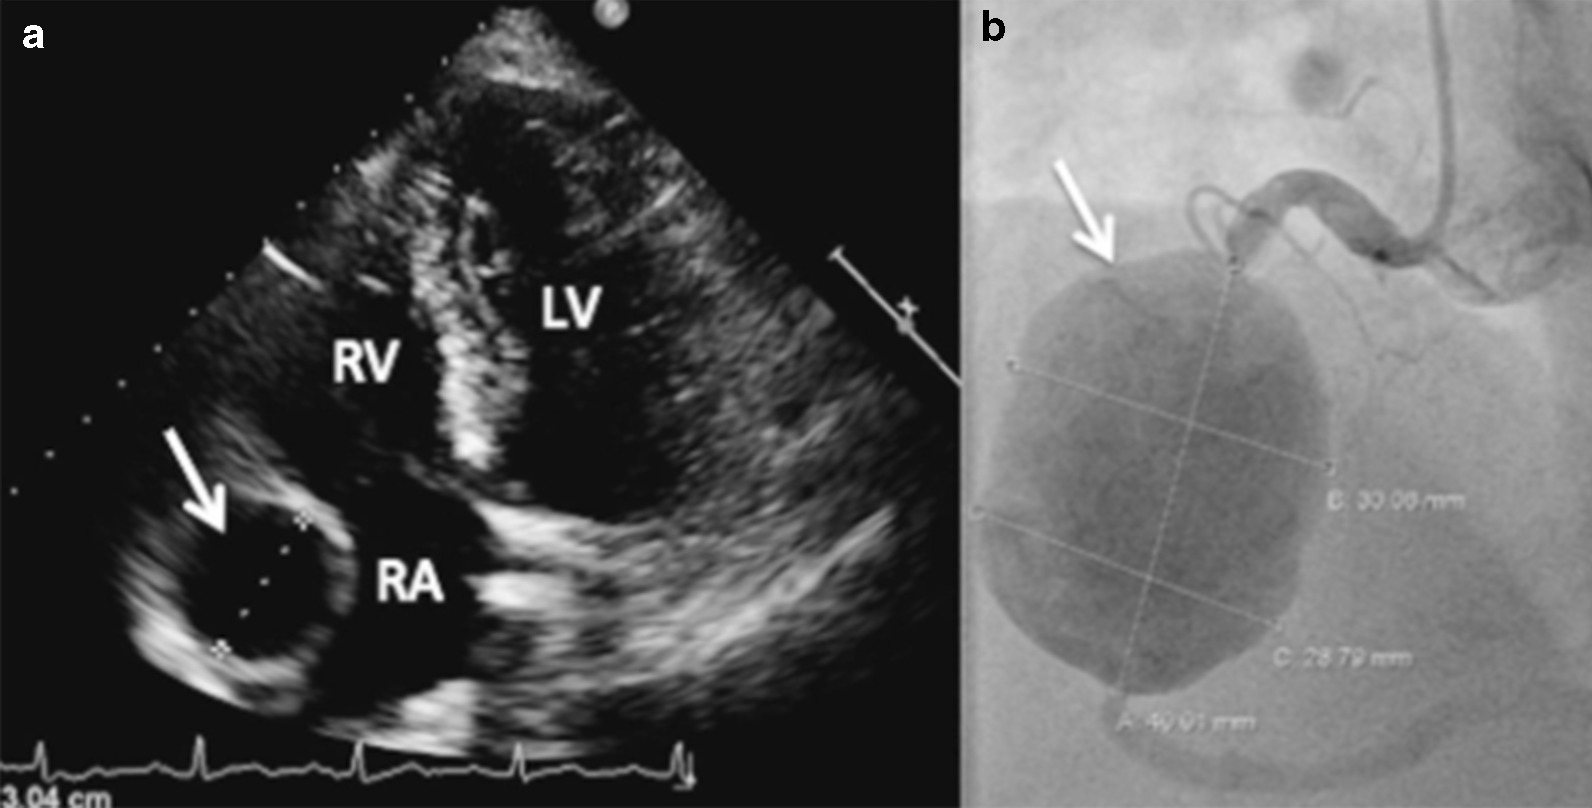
Echocardiogram shows a 30 mm wide hypoechoic structure lateral to the right atrium (arrow) (a). The structure is diagnosed as 30 × 40 mm right coronary aneurysm (arrow) using coronary angiography (b). (Adapted with permission from Ebersberger U, Rieber J, Wellmann P, Goebel C, Gansera B. Polyarteritis nodosa causing a vast coronary artery aneurysm. J Am Coll Cardiol. 2015 10;65(5):e1–2. https://doi.org/10.1016/j.jacc.2013.08.1667. PMID: 25660937) Copyright [2013] by Ullrich Ebersberger, JACC
